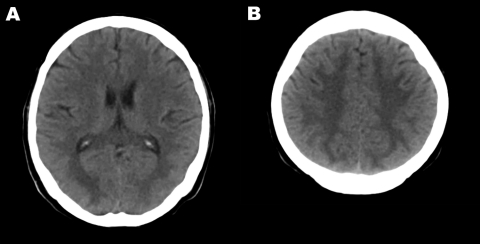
Computed tomography images of the brain of an adult patient with pandemic (H1N1) 2009 virus infection and neurologic signs. A noncontrast study showed hypodense lesions in both occipital lobes (A) and in both upper parietal lobes (B).
